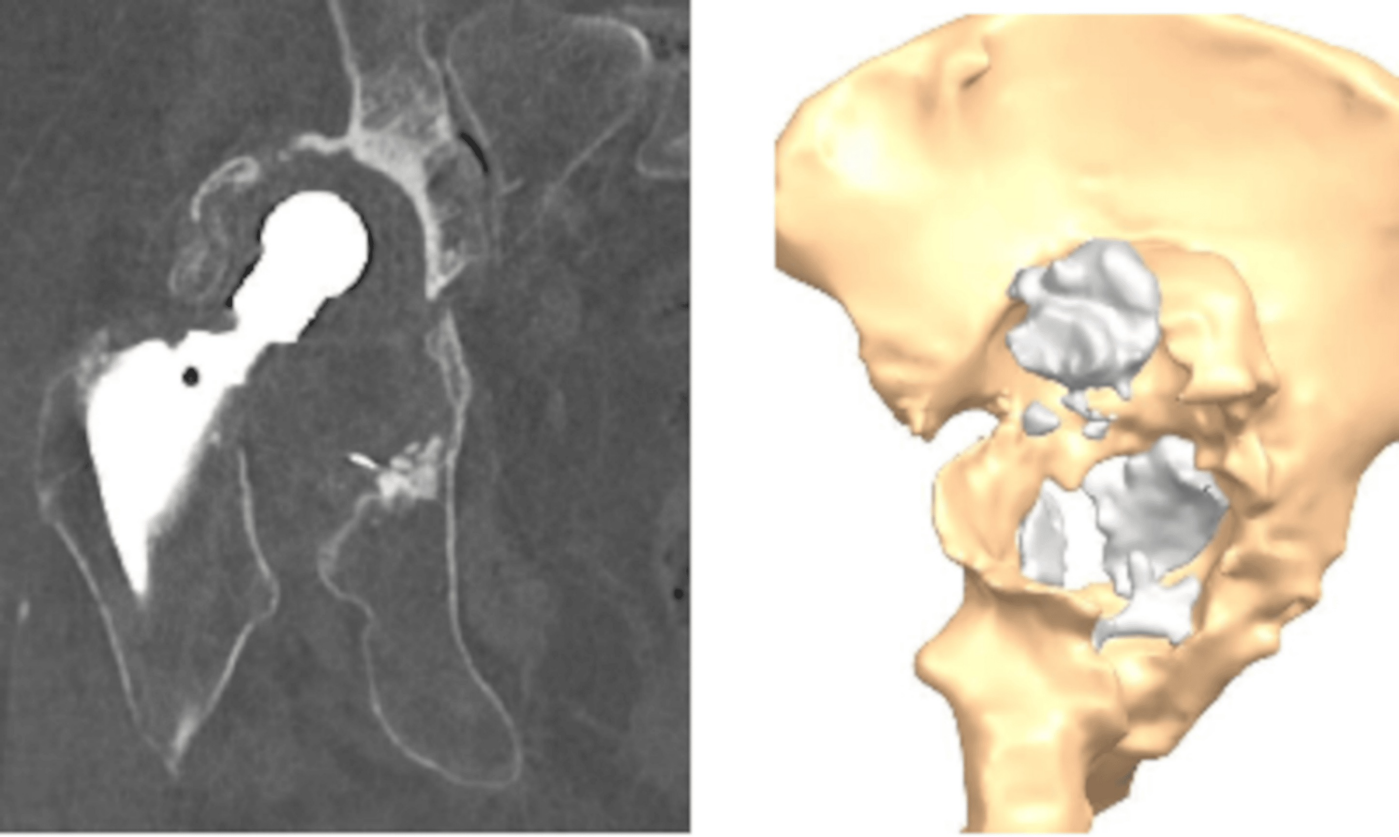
Thin-layer computed tomography with 3D reconstruction from February 14, 2022. CT scan quality leading to uncertainty in 3D reconstruction.
